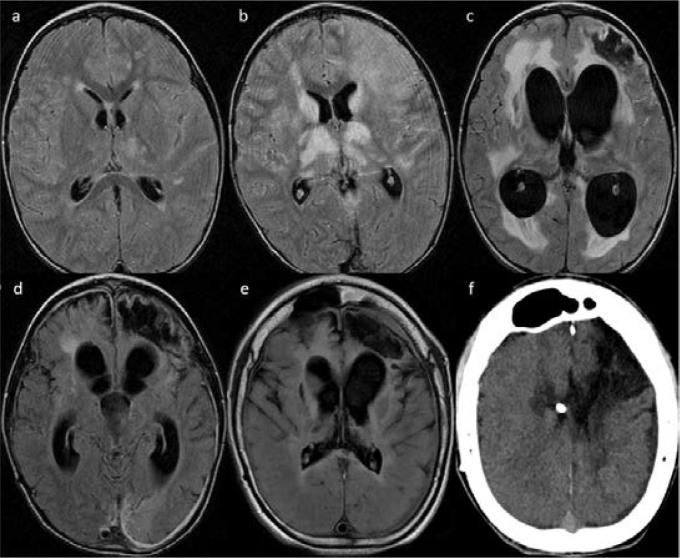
(A) magnetic resonance imaging (MRI) at admission. A few ischaemic foci are already observed as FLAIR-hyperintensities in both cerebral hemispheres. (B) MRI after 1 week (FLAIR). More extensive, confluent, bilateral infarcts. The biggest one, cortical–subcortical, involves the left frontal lobe. (C) MRI 1 month after disease onset (FLAIR). Hydrocephalus with CSF transudation, post-ischaemic lesion in the left frontal lobe with laminar necrosis of the overlying cortex. (D) MRI after 7 months (FLAIR). Progression of hydrocephalus, scarring of the right frontal lobe as well, subdural haematoma over the left cerebral hemisphere. (E) MRI after >6 years (FLAIR). Smaller degree of ventricular dilatation after ventriculoperitoneal shunt placement. Abnormal, hyperintense white matter with lacunar post-infarct foci. Cortical–subcortical scars in both frontal lobes (L > P). Chronic bilateral subdural haematomas. (F) CT after >12 years. Overdrainage with slit-like lateral ventricles. Scar in the left frontal lobe. Thickened calvarial vault associated with ventricular shunting for hydrocephalus at a young age was observed 6 years earlier (G).
